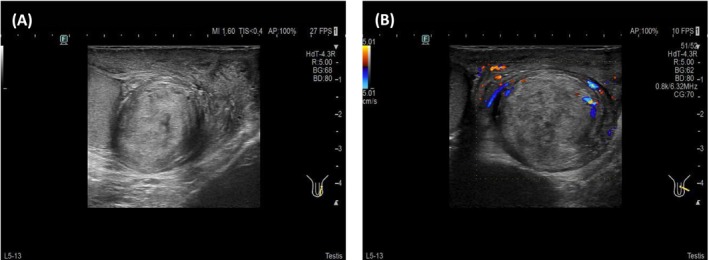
Ultrasound of paratesticular adenomatoid tumor. (A) Grayscale ultrasound showed an irregular, well‐defined, heterogeneous, and hyperechoic mass located at the caudal aspect of the left epididymis. (B) Color Doppler flow imaging showed short, rod‐like vascular signals both within and adjacent to the mass.
